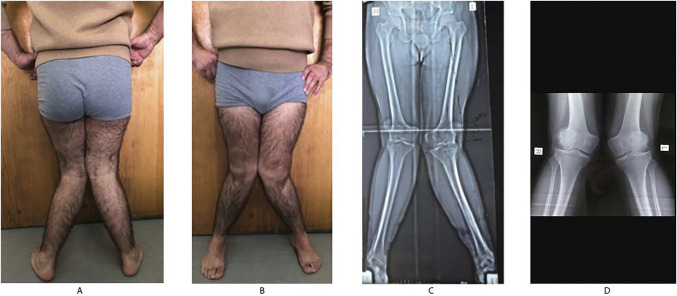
A and B, Clinical images showing the anterior and posterior pre-reconstruction. C, The preoperative standardized long leg standing panoramic radiograph of the lower limb was done for frontal plane analysis. The hip-knee-ankle angle on the right side was 28° and 30° on the left side. D, Short radiographs show the level II osteoarthritis on the lateral compartment of the knee.
